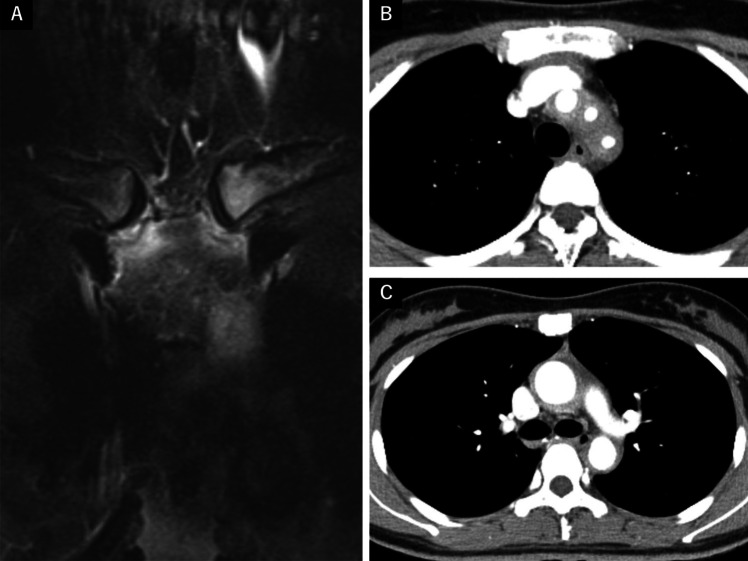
A Chest magnetic resonance imaging showing high-intensity areas in the sternoclavicular and sternocostal joints. B C Chest computed tomography demonstrating circumferential thickening with a contrast effect of the ascending to descending aorta, bilateral common carotid arteries, and the left subclavian artery
